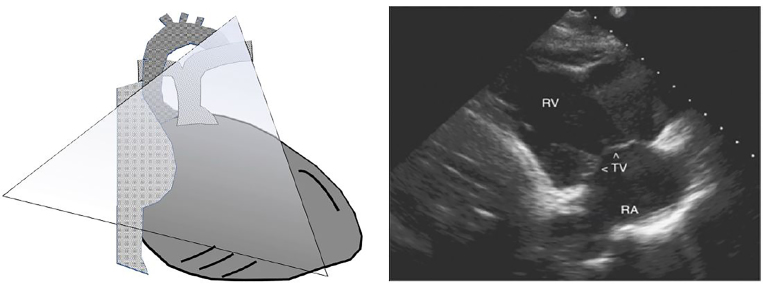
Right ventricle inflow tract view. Left: Graphical representation. Right: Point-of-care ultrasound with normal anatomy. *RA*, right atrium; *RV*, right ventricle; *TV*, tricuspid valve.
